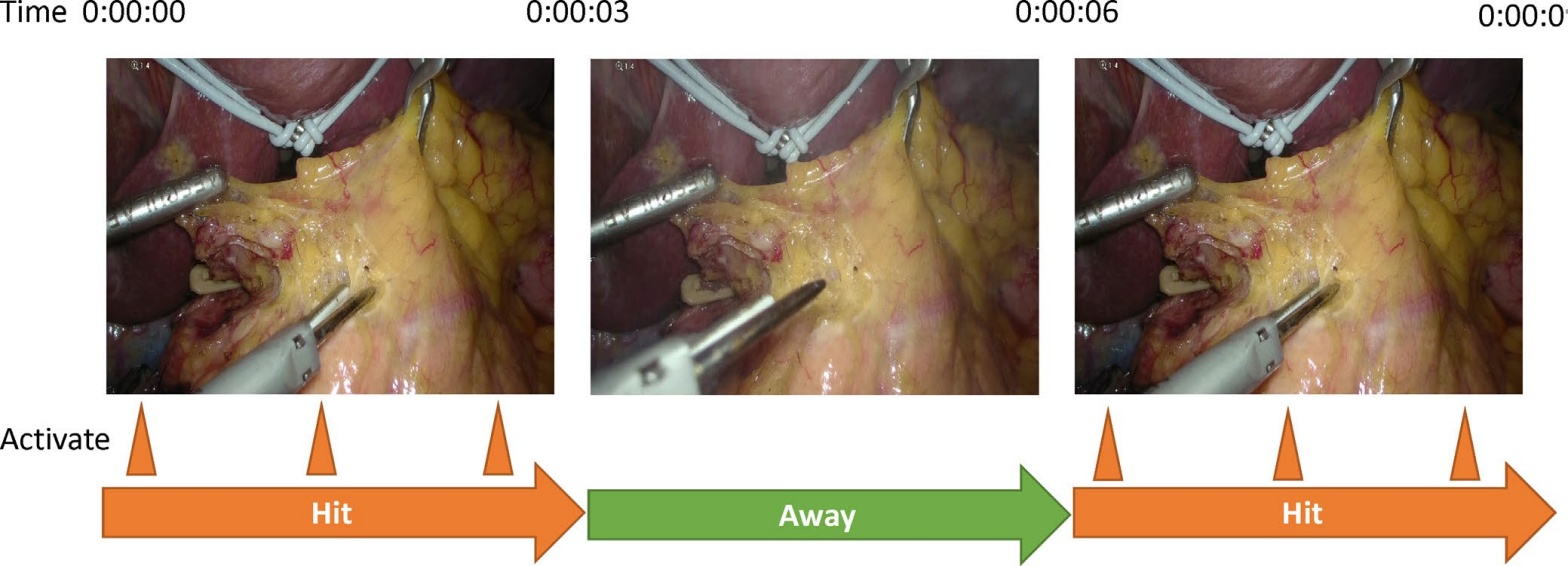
‘Hit and Away’ technique. In the ‘‘Hit’’ phase, surgeons perform three activations with the tip of the ultrasonic scalpel after the tissues and vessels are clamped in a block. After three activations, the ultrasonic scalpel is immediately released. These figures were cited from Irino et al (2016)^57^
